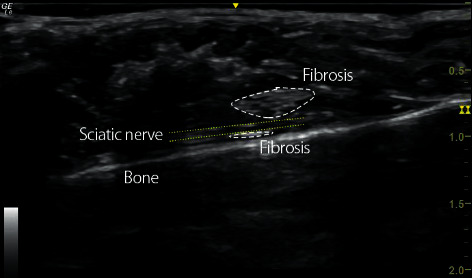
Nerve ultrasonography. Ultrasound imaging (longitudinal *B*-mode) shows sciatic nerve (yellow discontinue lines) with surrounding fibrosis (white discontinue lines).
